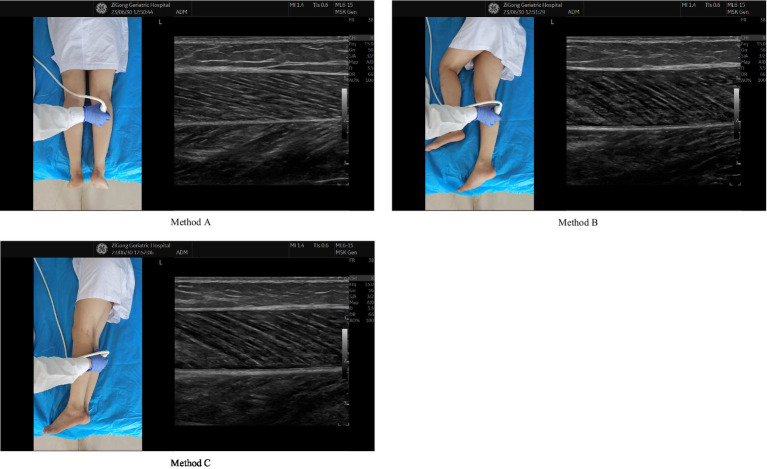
Three different sonographic protocols. Method A lying prone on the examination bed with both legs straight and relaxed and both feet hanging outside the examination bed. Method B lying on the right side with the left leg flexed and the right leg relaxed. Method C lying on the right side with legs together with the lower body muscles in a relaxed state.
